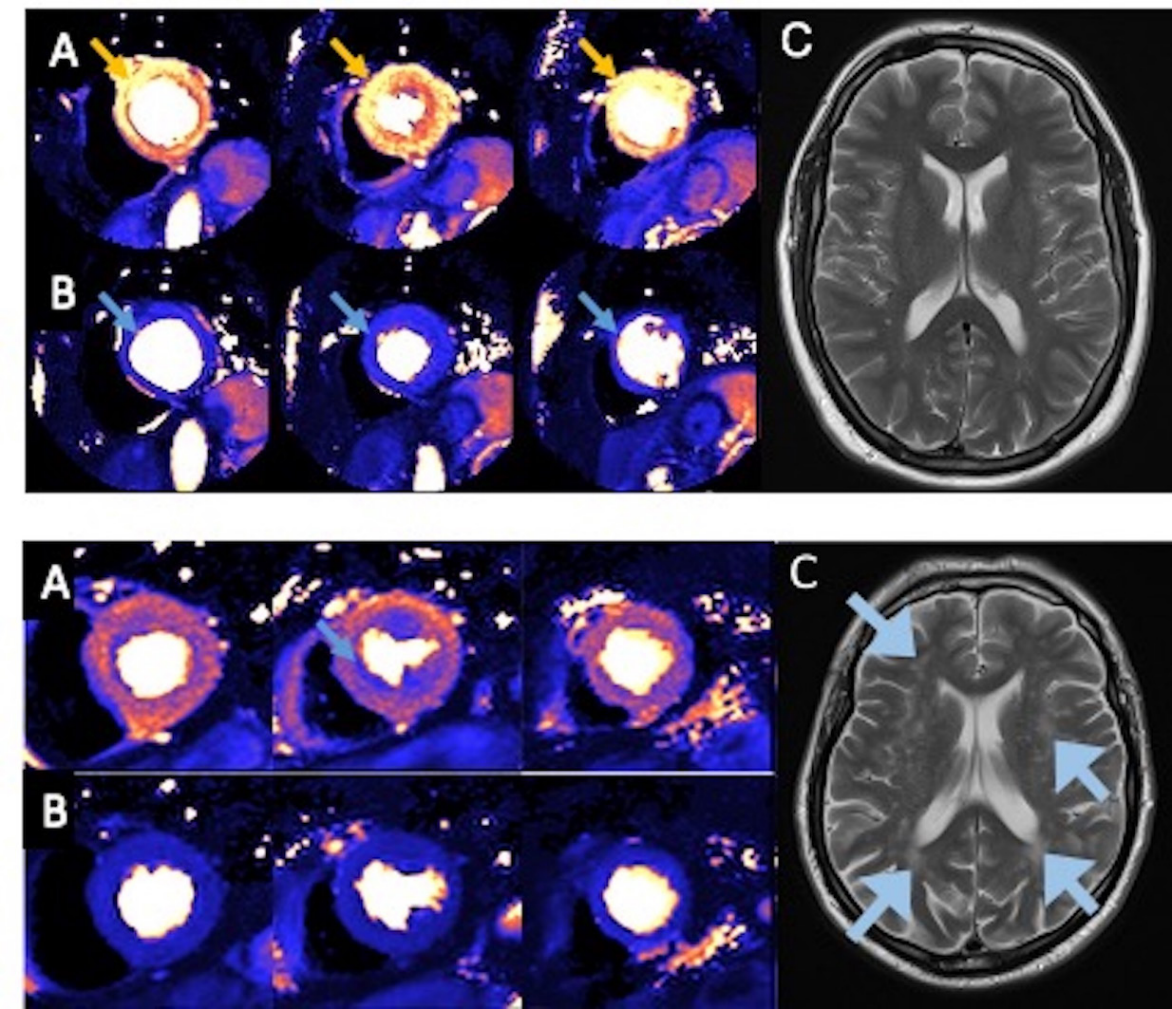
Two clinical cases from coronary microvascular angina cardiovascular magnetic resonance imaging (CorCMR) Trial. Top: normal cardiac and brain MRI scans. A 42-year-old man with anginal symptoms and no obstructive coronary artery disease on invasive coronary angiography. He was enrolled into the CorCMR clinical trial. (A) Normal stress MRI (bright orange, arrow) with no perfusion defects (ie, no residual blue colour). (B) Cardiac MRI shows normal myocardial blood flow at rest (uniform dark blue, arrow). (C) Normal MRI brain scan with no evidence of cerebral small vessel disease (CSVD). Bottom: small vessel disease in the heart and brain. A 59-year-old woman with anginal symptoms and no obstructive coronary artery disease on invasive coronary angiography. She was enrolled into the CorCMR clinical trial. (A) Stress MRI showing circumferential subendocardial perfusion defect (dark blue, arrow) that contrasts with the normal hyperaemic blood flow (bright orange) elsewhere in the heart. Diagnostic of coronary microvascular dysfunction/microvascular angina. (B) Cardiac MRI shows normal blood flow at rest (upper panel – dark blue, arrow). (C) Brain MRI showing extensive periventricular and subcortical white matter hyperintensities (blue arrows) in keeping with CSVD. In summary, this participant has microvascular angina and brain imaging features of CSVD.
